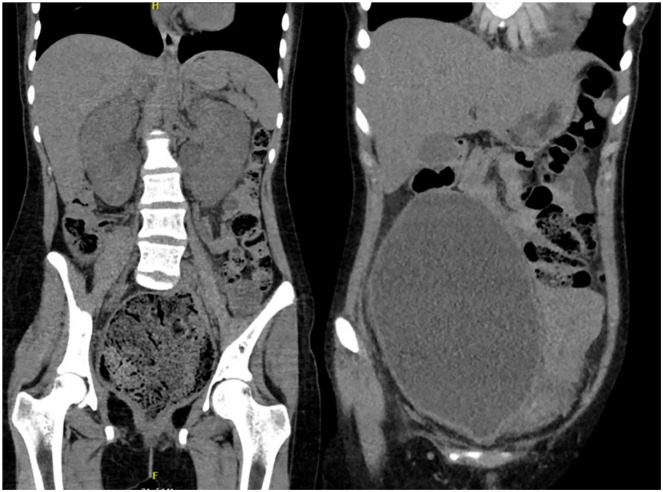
A coronal CT showing a rectal fecaloma (left) causing urethral obstruction and a massively dilated urinary bladder (right).
